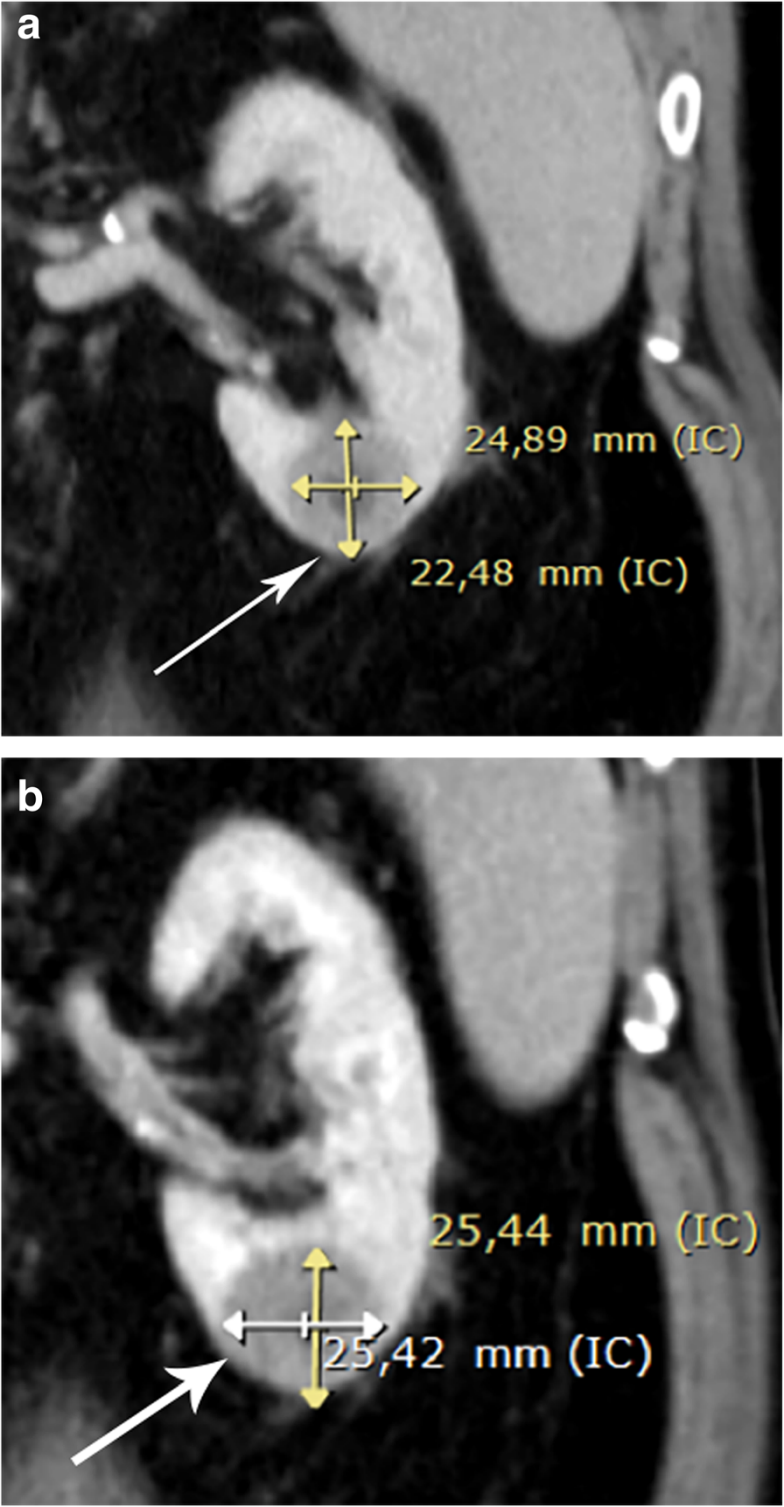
Calculation of the linear growth rate (GR) of a lower third left kidney small renal mass (SRM) undergoing active surveillance (AS) in a 65-year-old patient (arrows). a Coronal contrast-enhanced CT of the renal mass showing an axial MTD of 2.5 cm (24.89 mm) at the beginning of the surveillance and (b) 3 which had only increased to 2.5 cm (25.44) 3 years later. With the use of the linear GR formula in centimeters, 2.5 cm − 2.5 cm = 0 cm divided by 36 months and multiplied by 12, the annual GR was shown to be 0 cm/year, indicating that the renal mass was stable. After 5 years of follow-up, the interval between imaging tests can be lengthened or even discontinued according to the decision of the multidisciplinary committee
